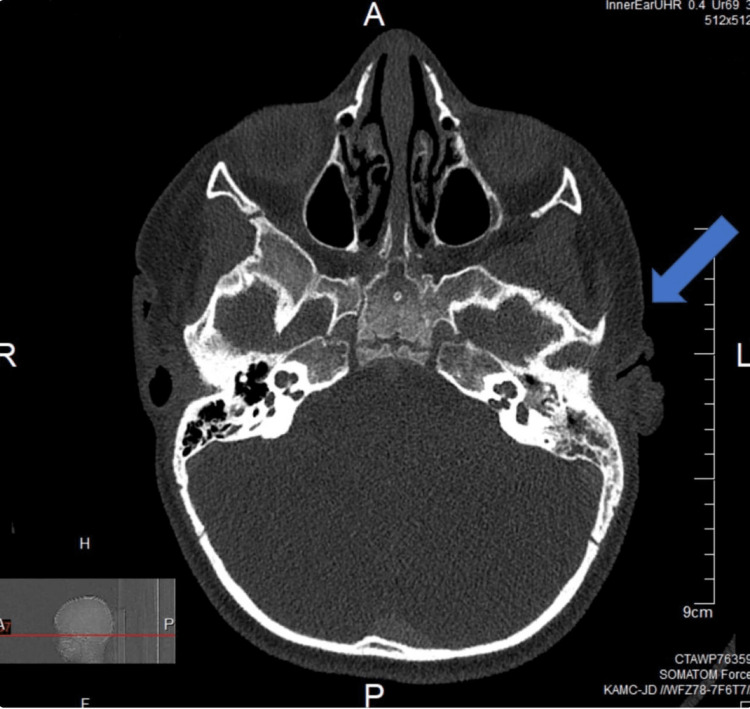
A preoperative CT scan of the mastoid at the level of the mesotympanum showing the opacification of the middle ear involving the sinus tympani, mastoid air cells, as well as the facial recess.
